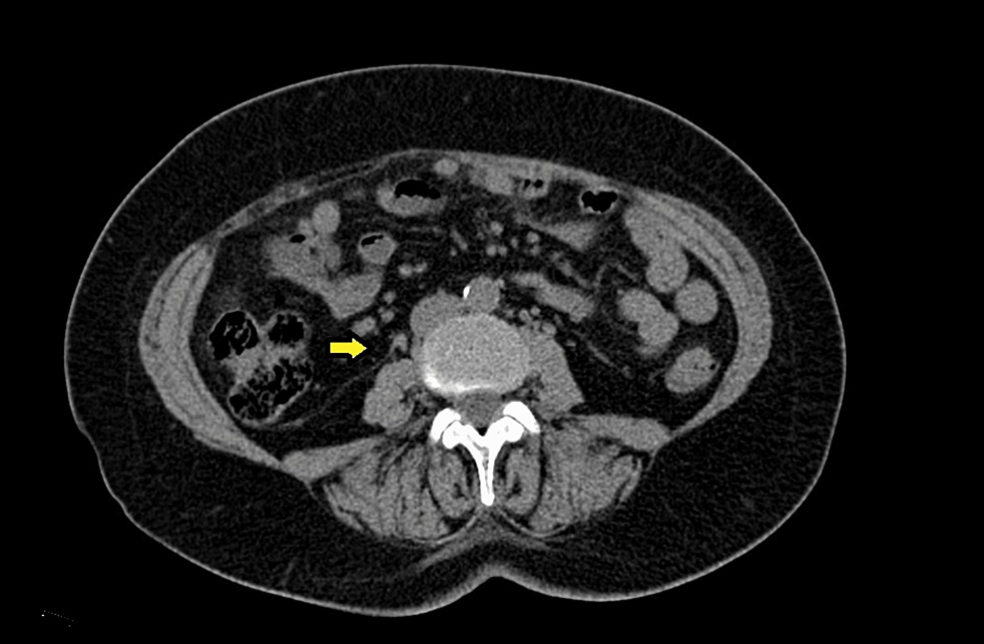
Follow-up CT scan showing smaller and resolving lymphadenopathy (yellow arrow), which were previously noted on the PET scan. CT: computed tomography, PET: positron emission tomography
